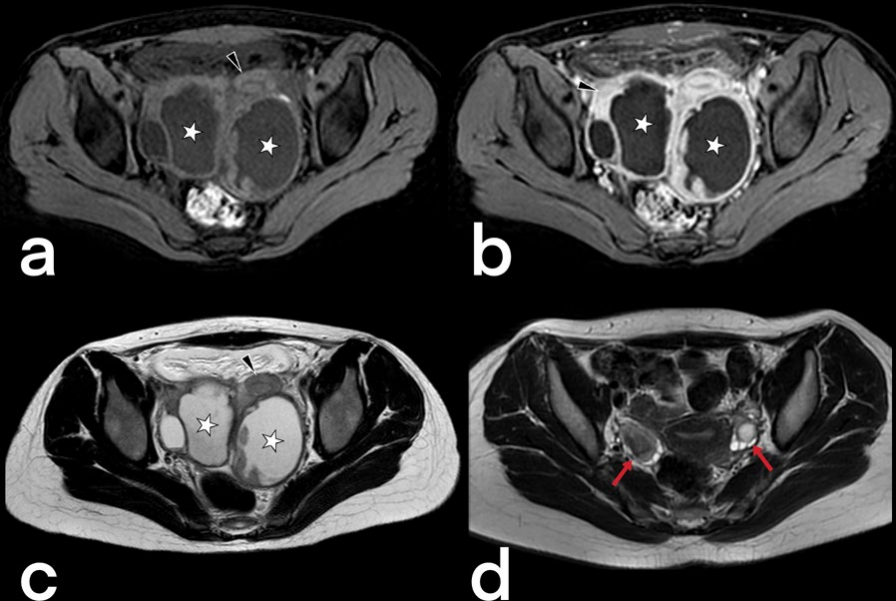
Bilateral tubo-ovarian TB in a 15-year-old-female with pelvic pain. Axial pre-contrast T1 weighted (a), post-contrast T1 weighted (b), and T2 weighted (c) MR images show bilaterally enlarged ovary (stars) and dilated fallopian tubes (arrowheads). On T2-weighted MR image obtained after antituberculous therapy of 10 months, bilateral ovaries (arrows) and fallopian tubes (not shown) appear normal (d)
